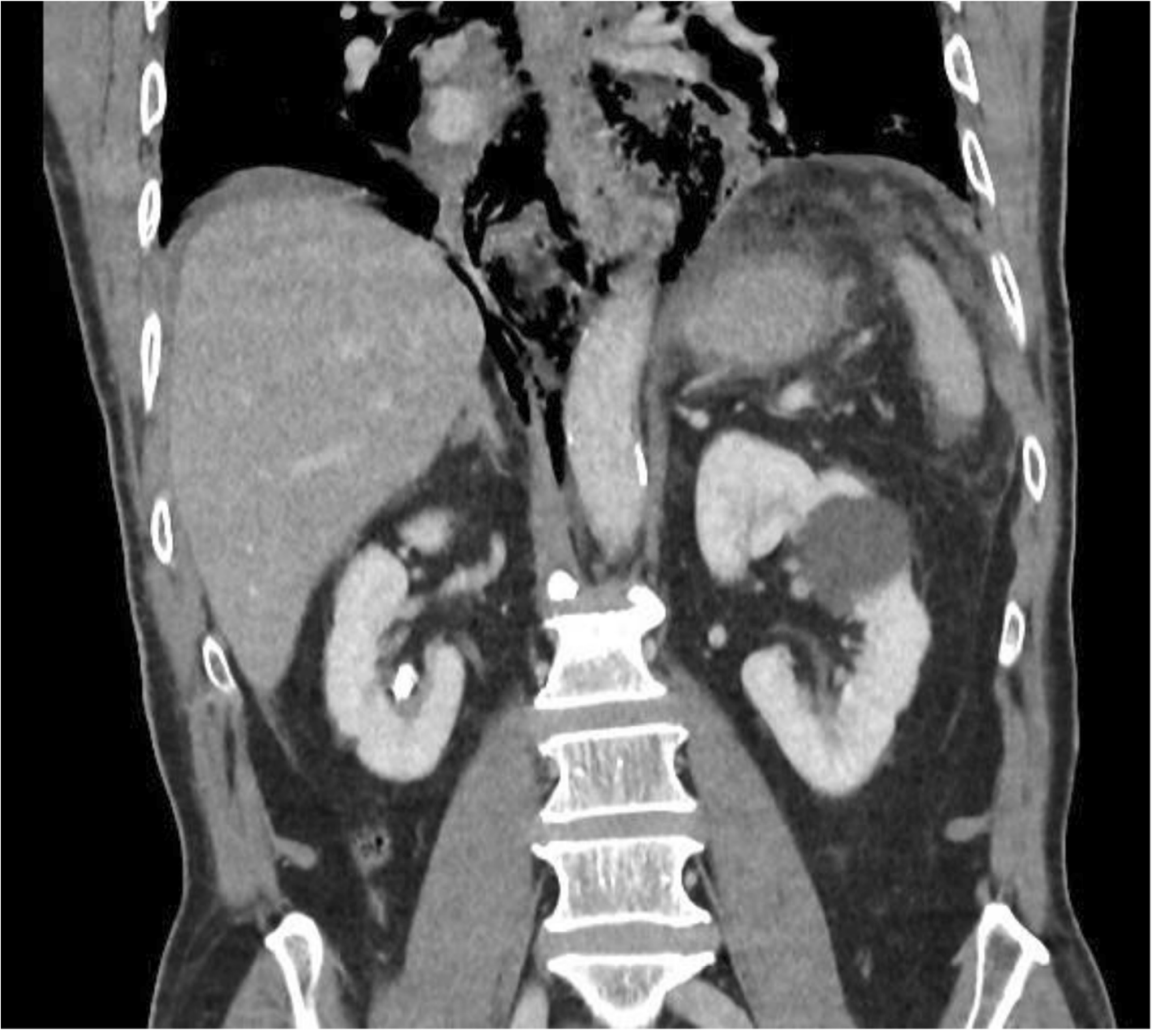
Coronal CT showing mediastinal air but minimal pleural reaction in a patient with spontaneous EP (Boerhaaves). The patient was successfully managed via laparotomy alone and transhiatal repair. Primary suture repair with interrupted full-thickness single-layer polyglycolic acid and fundoplication healed without a leak
